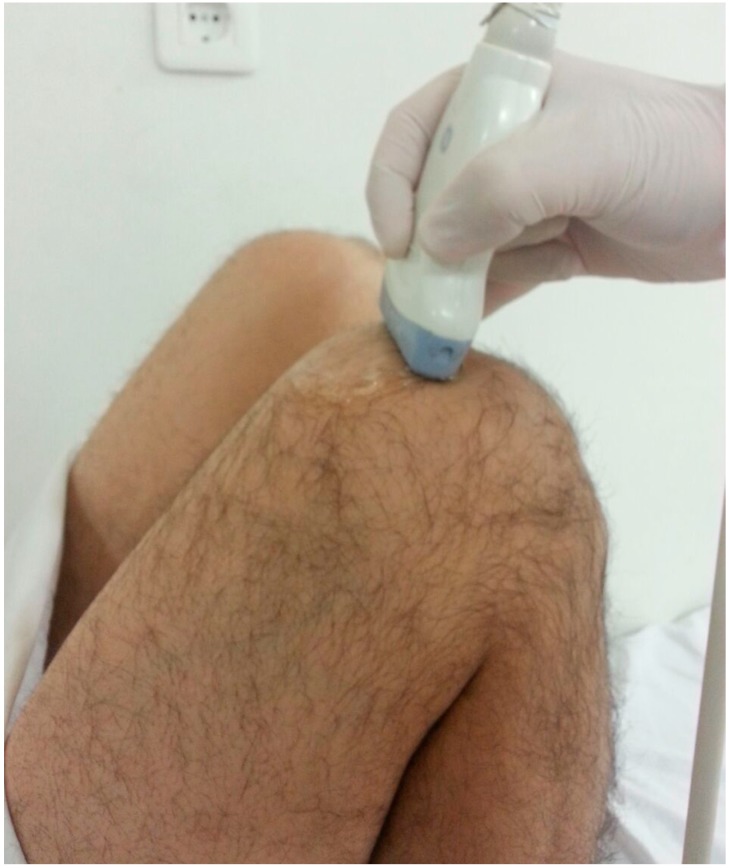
Experimental setup position of the knee and the ultrasound probe.
